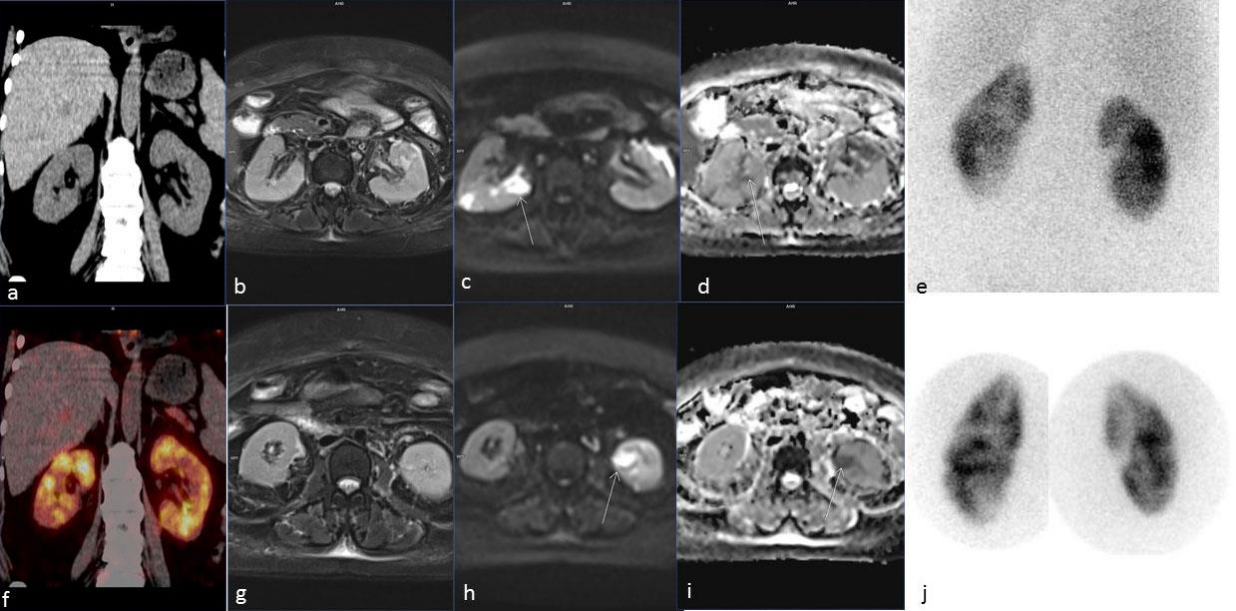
Coronal CT (a) and fusion ^68^Ga-FAPI PET CT (f) images show diffuse increased tracer uptake in both kidneys. The T2-Weighted sequence of MRI (b and g) shows increased cortical-based signal intensities. Diffusion-weighted images show hyperintense signals in high B (c and h) appearing dark on apparent diffusion coefficient maps (d and i). Dimercaptosuccinic acid planar images with LEHR (e) and pinhole (j) collimators show the normal size of both kidneys with cortical irregularities and multiple cortical-based areas of photon deficiencies compatible with pyelonephritis
